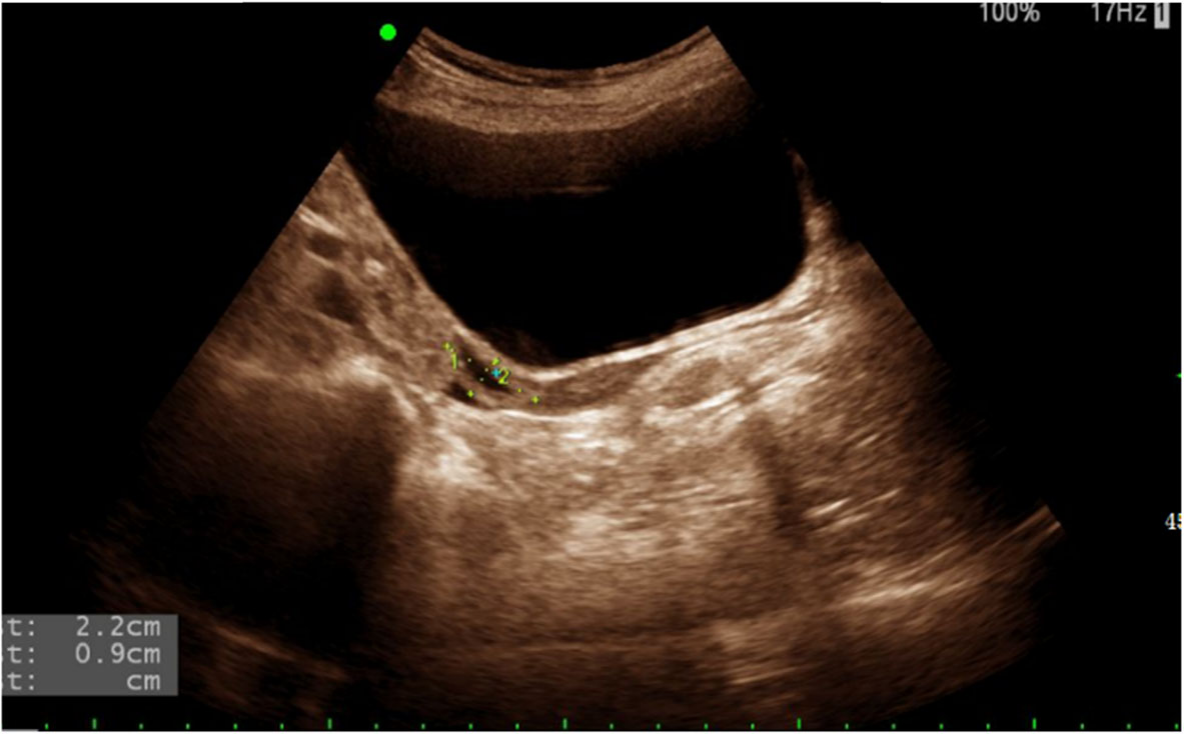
Pelvis ultrasonography of the patient. It showed immature uterus, not detecting bilateral ovaries
